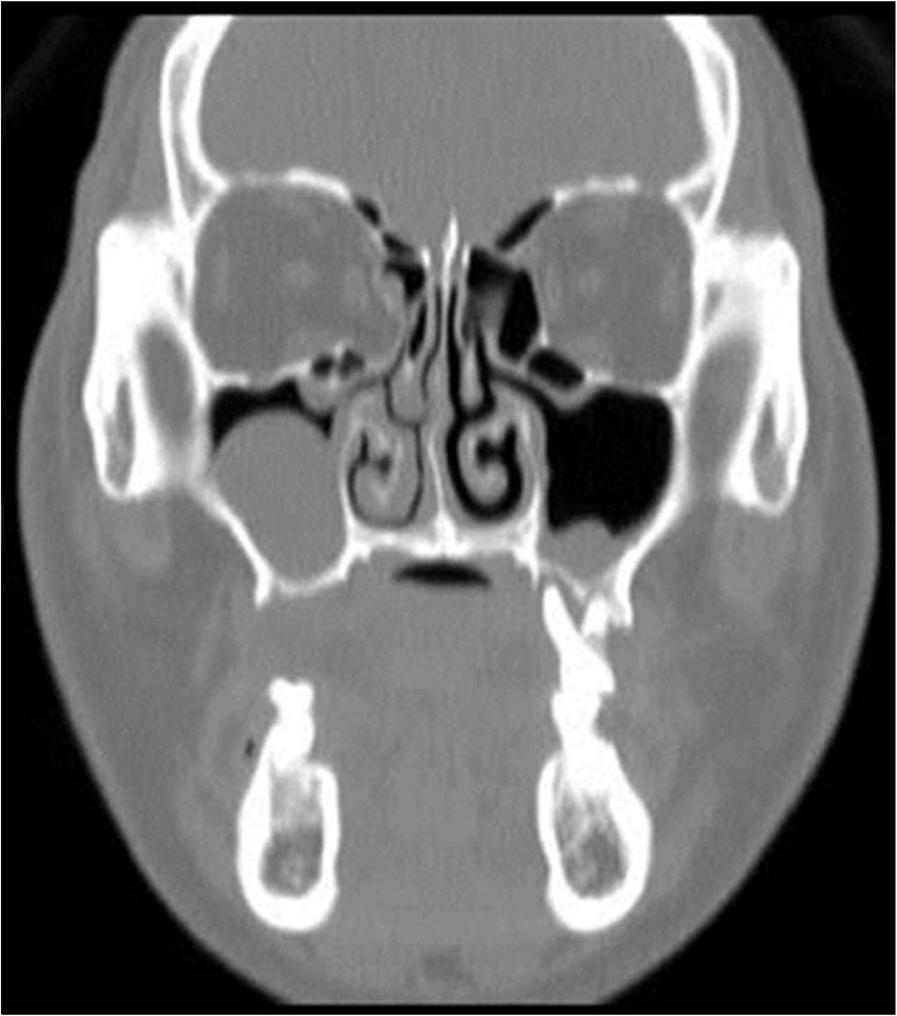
Preoperative PNS CT view. Dome-shaped radiopacity is observed in the right maxillary sinus
